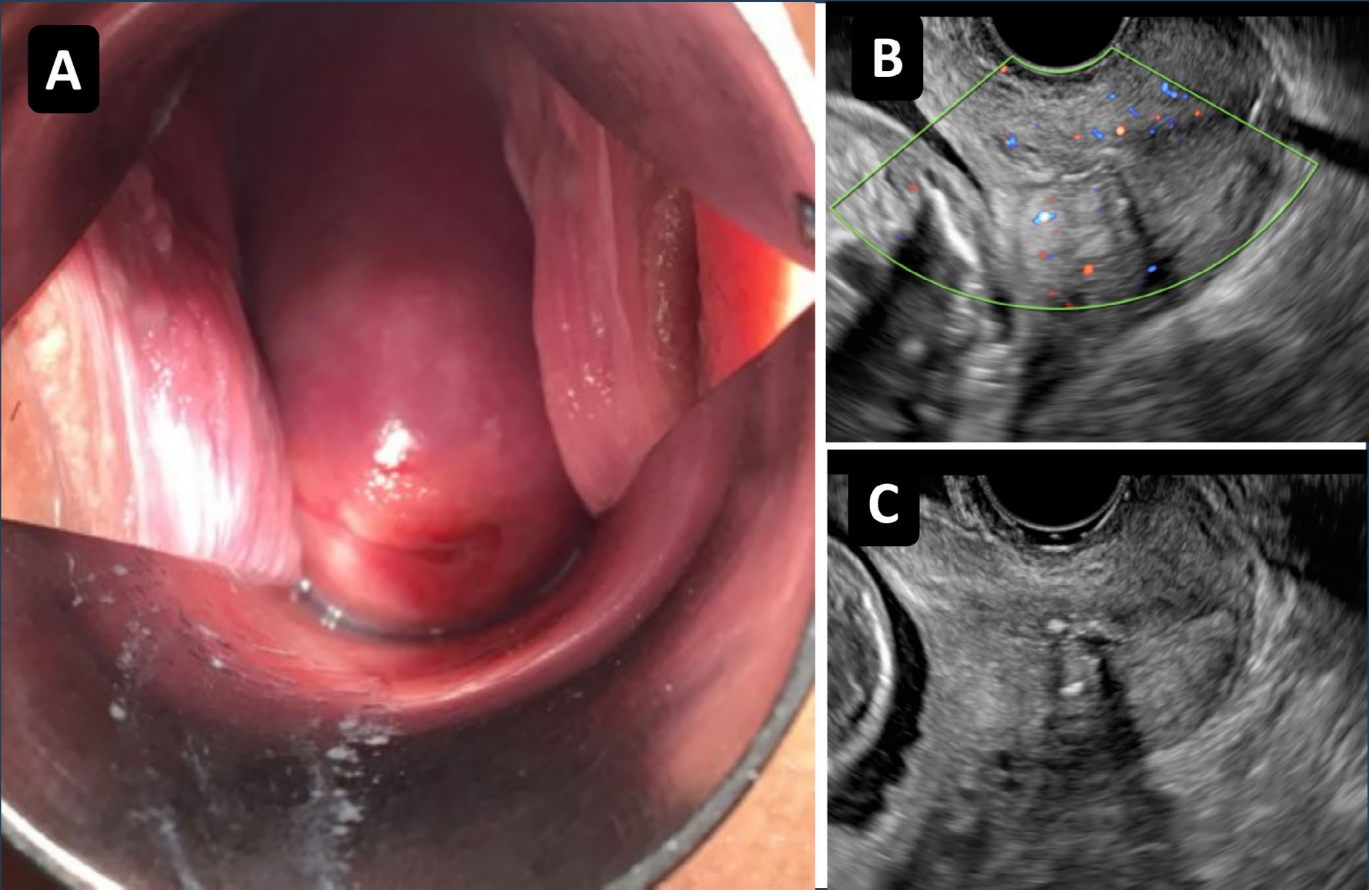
Follow‐up finding after complete resolution of the cervical polyp. (A) Complete disappearance of the cervical polyp at 14 weeks and 6 days of gestation. (B) Doppler ultrasound showing absence of blood flow in the cervical canal. (C) Triclosan‐coated suture remaining in situ within the cervix.
